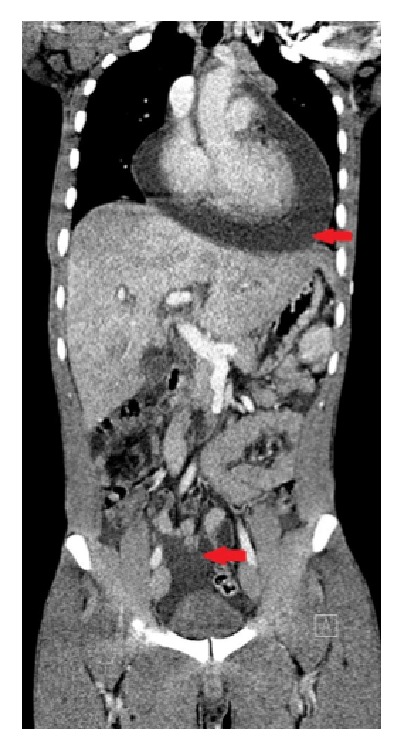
Computed tomography (CT scan).* Fluid accumulation within the pericardium and abdomen (red arrows).
